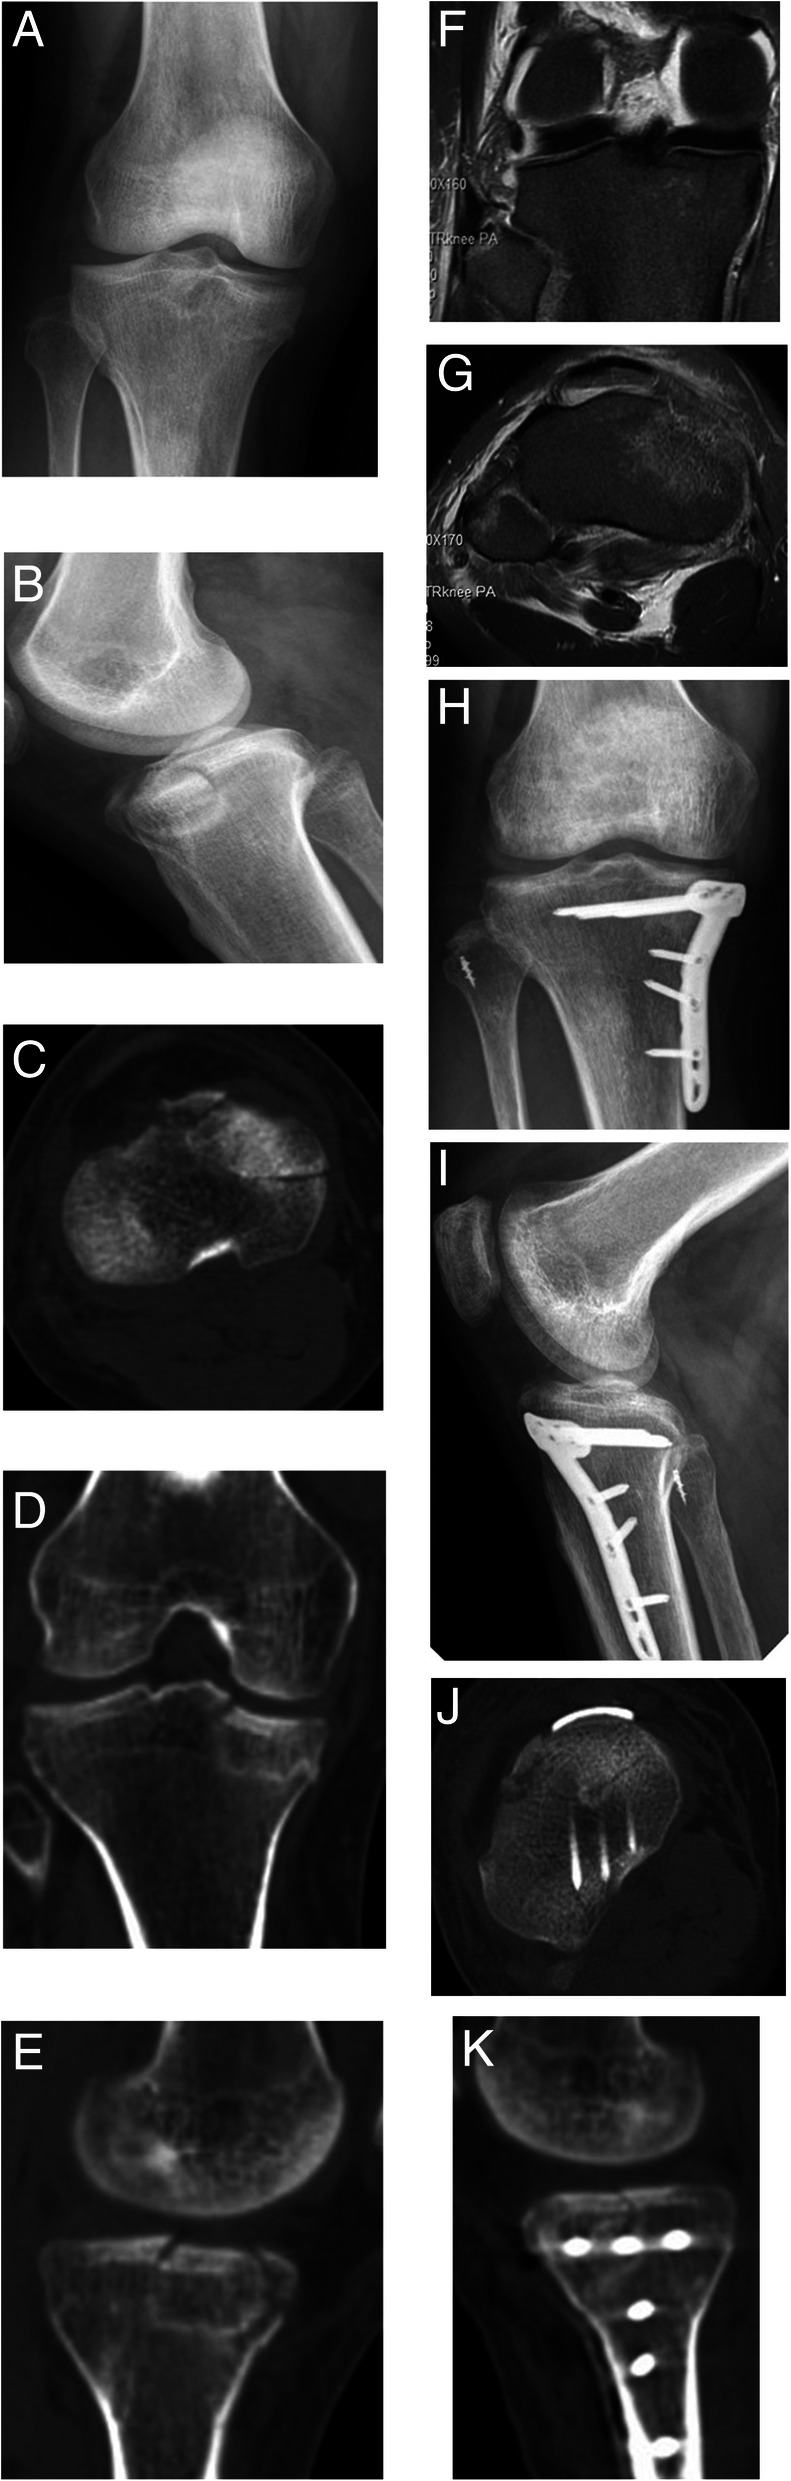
M, 36Y, anterior medial tibial plateau fracture with PLC injury. a, b Preoperative x-ray shows anteromedial tibial plateau fracture. c–e Preoperative CT shows collapse of anteromedial tibial plateau with significant change of pTSA. f, g Preoperative MRI showed posterolateral angle injury and partial tear of lateral collateral ligament at fibula. h–k Postoperative x-ray and CT showed the anatomical tibial plateau fracture reduction, and the lateral collateral ligament was repaired with a suture anchor
